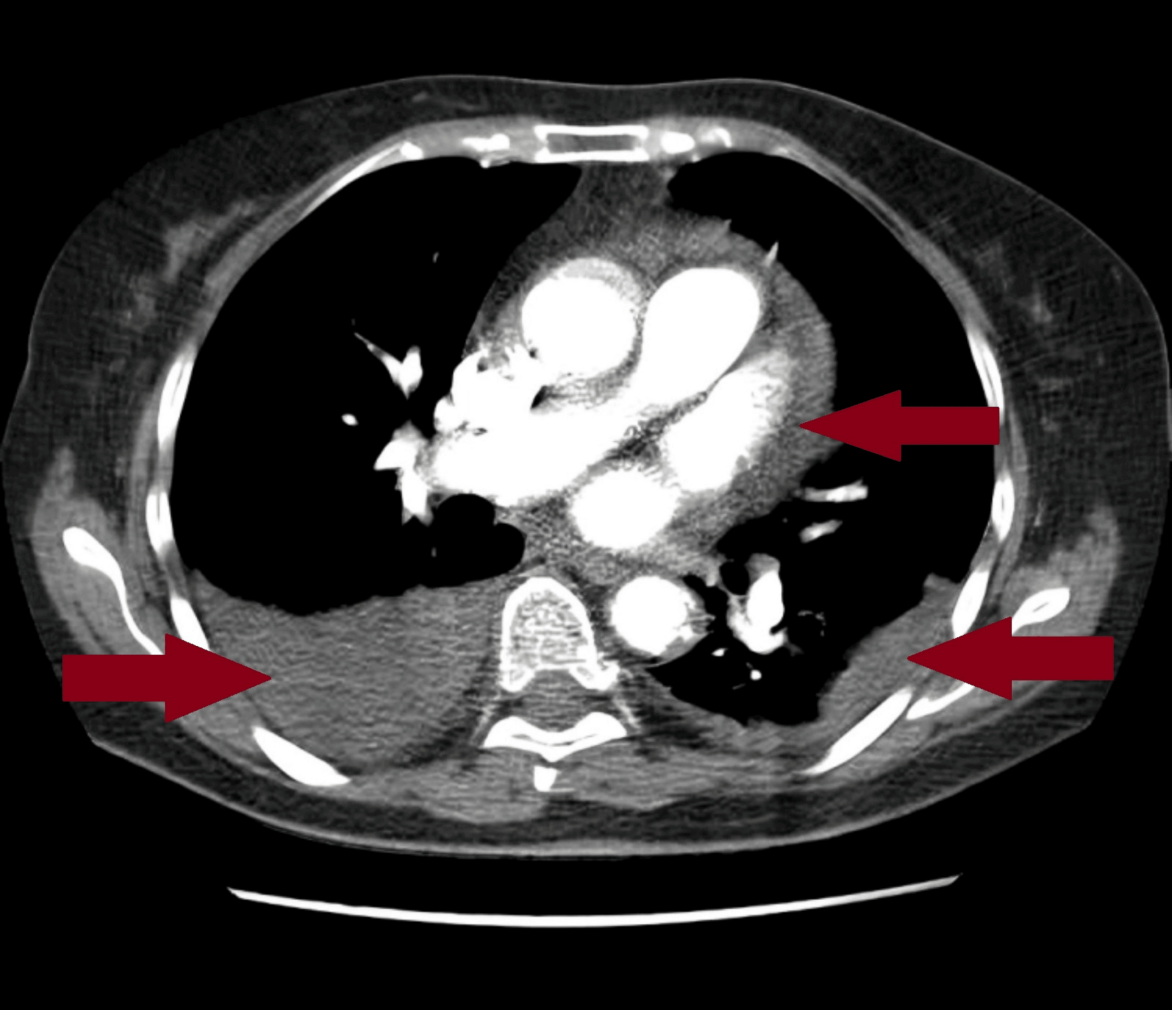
Admission CT A pericardial effusion 10 mm in diameter, a left-sided pleural effusion 15 mm in diameter, and a right-sided pleural effusion 38 mm in diameter are seen. CT: computed tomography
